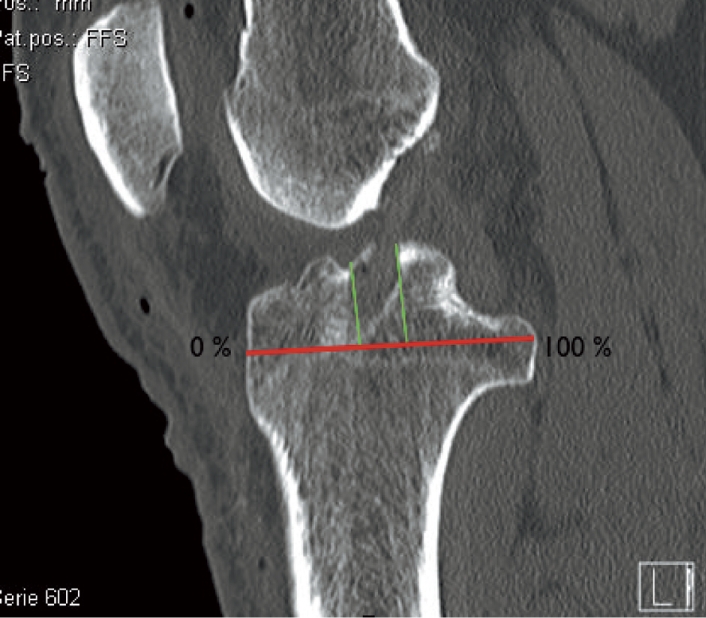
Measurement according to Stäubli (Stäubli and Rauschning, 1994) on the sagittal tibial CT reconstruction. The aperture of the tibial tunnel is compared to the line parallel to the joint line, through the most anterior and posterior points of the intracondylar tibial plateau.
